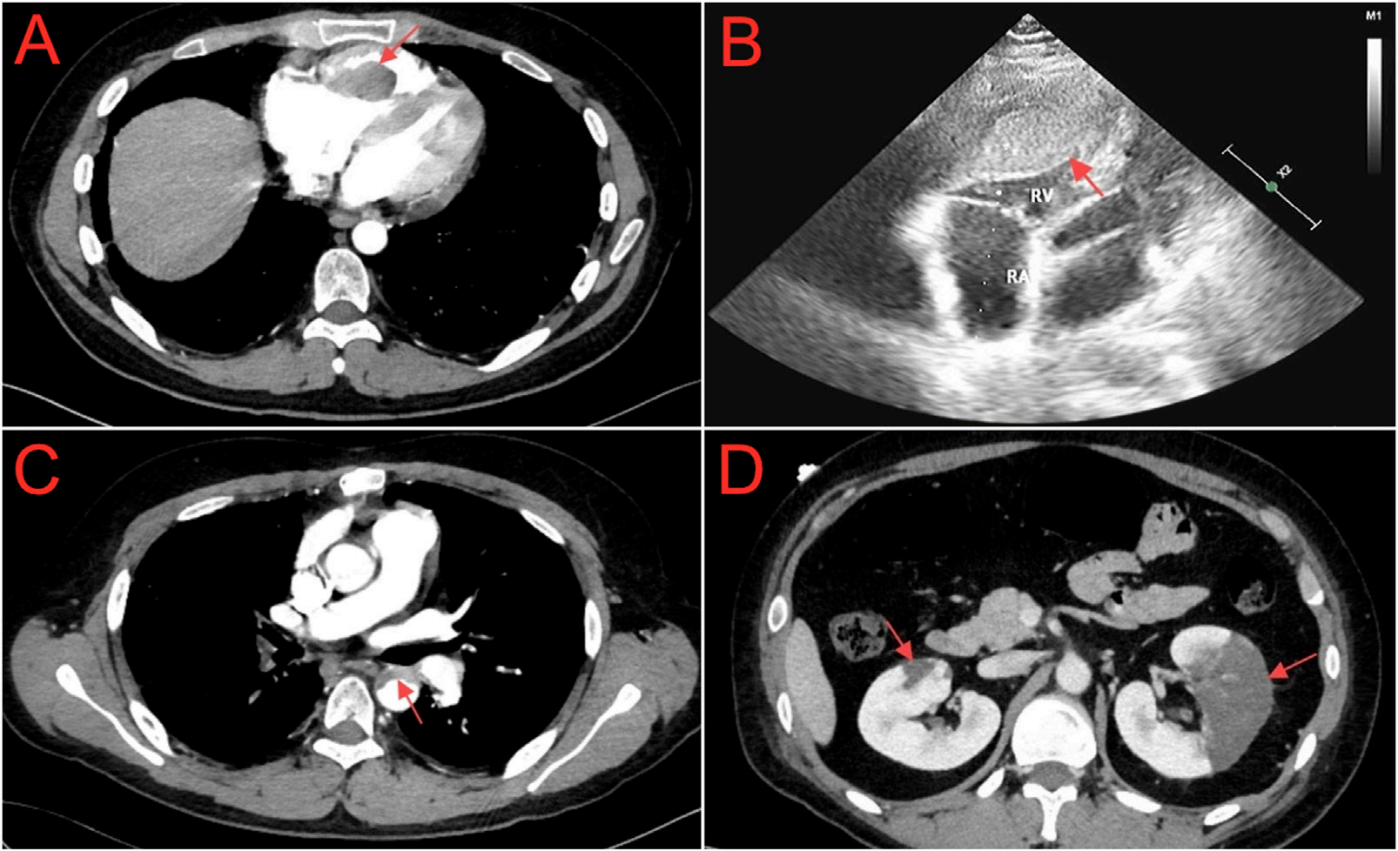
(A) Chest computed tomography angiography shows filling defect in the right ventricle (red arrow). (B) Echocardiogram shows a slightly hypoechoic area within the right ventricular cavity, with dimensions of approximately 32.4 × 20.9 × 61.9 mm (red arrow). (C) Abdominal computed tomography angiography shows a curved, non - enhancing, slightly low - density shadow within the lumen of the descending aorta (red arrow). (D) Abdominal computed tomography angiography shows irregular sheet - like low - density opacities in the renal parenchyma, notably more pronounced in the left kidney (red arrow).
